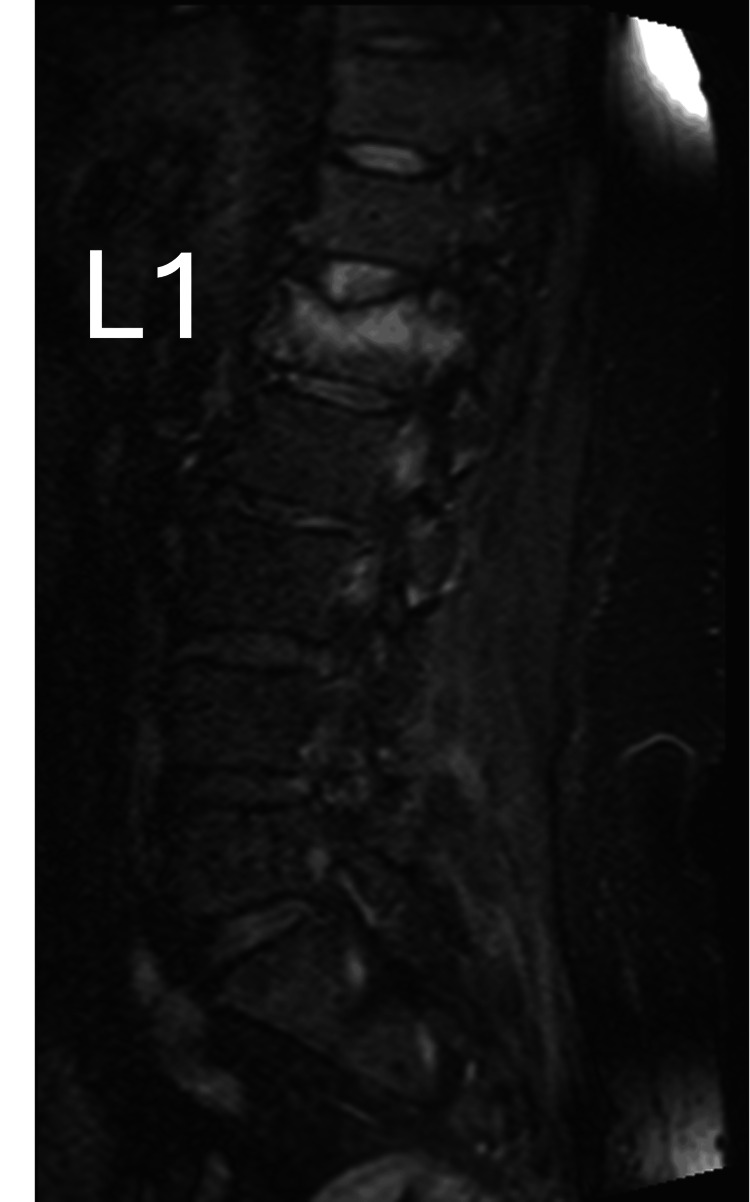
MRI TIRM T1 weighted image (sagittal view) of the lumbar spine obtained immediately following the fracture showing the edema of the vertebral body of L1. TIRM: turbo inversion recovery magnitude
